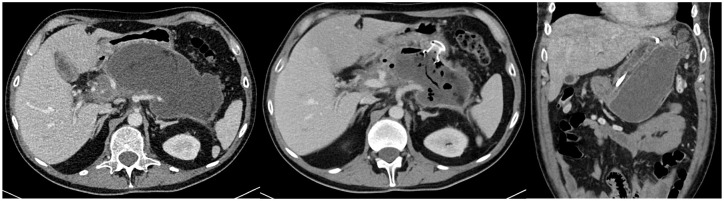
(Left) CT scan with large WON. (Middle) CT scan of the same patient as left after HotAxios implantation. (Right) CT scan of PPC (inlaying nasogastral tube). CT, computed tomography; PPC, pancreatic pseudocyst; WON, walled-off necrosis.
